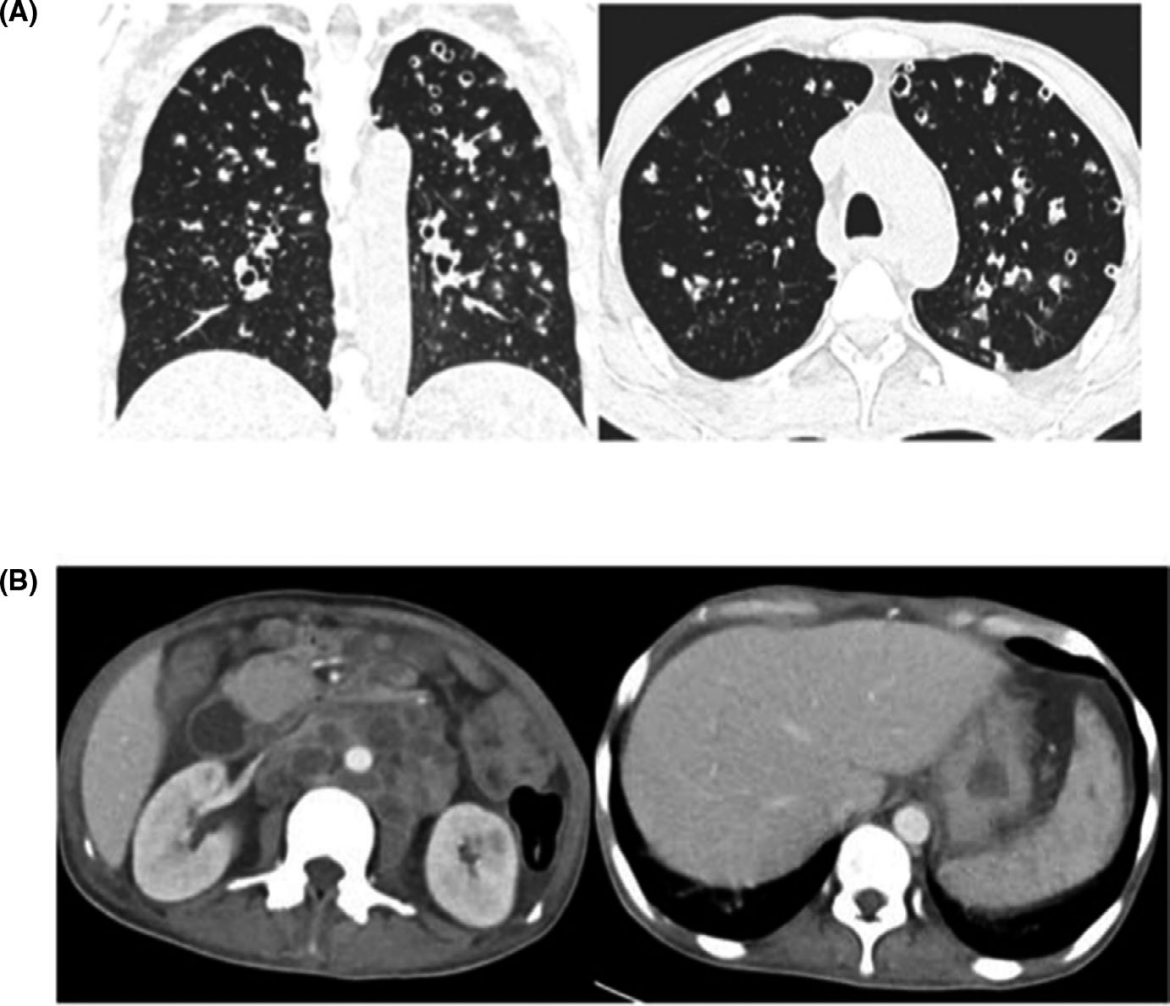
A: Atypical CT imaging features for COVID‐19. Unenhanced axial and coronal CT images showing diffuse tree‐in‐bud nodules with central cavitation. B, Contrast‐enhanced CT images of the abdomen and pelvis showing multiple necrotic lymph nodes with multifocal hypodense lesions in the spleen
